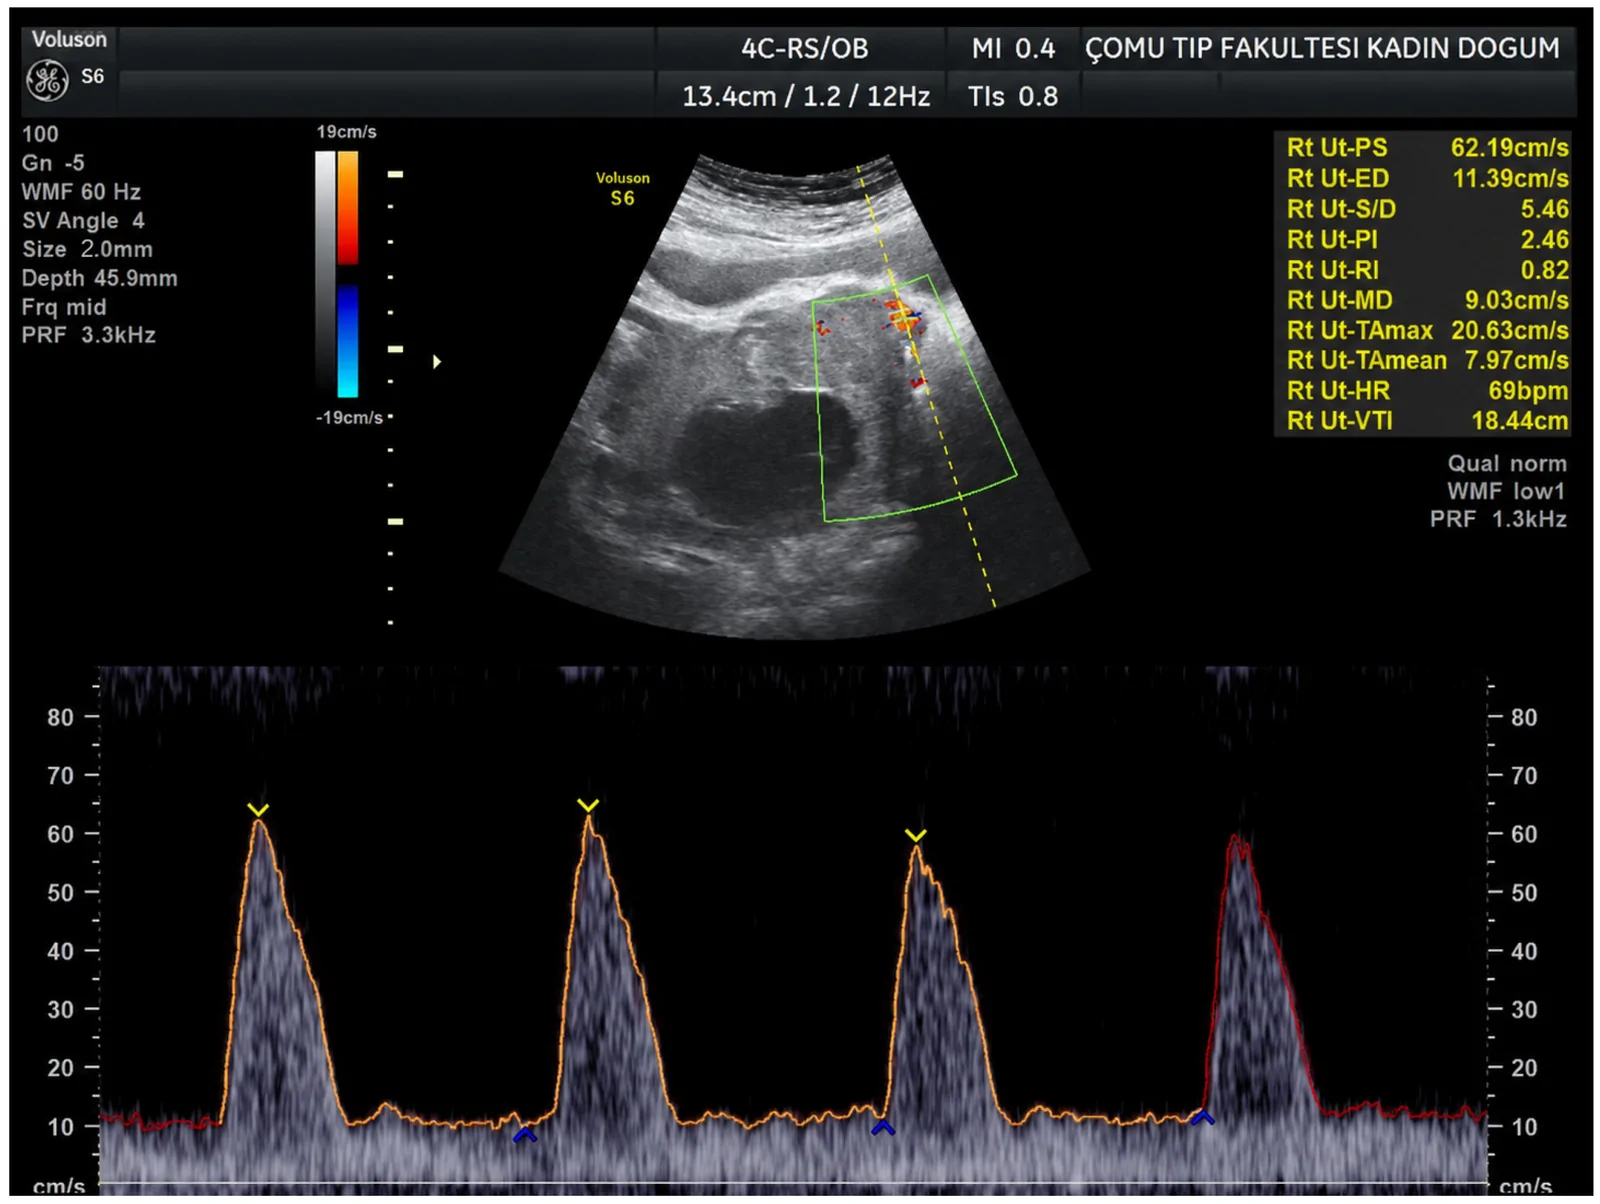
Representative bilateral uterine artery Doppler examination performed according to the Fetal Medicine Foundation protocol. The three orange-traced waveforms represent the measured cycles used for pulsatility index calculation; the additional (red-traced) waveform is an adjacent, unmeasured cardiac cycle automatically displayed by the ultrasound system. The green box denotes the Doppler sample gate positioned over the uterine artery, the yellow dashed line indicates the angle-correction line used to align the insonation angle with the vessel, and the yellow and blue chevrons mark the automatically identified systolic peak and end-diastolic points, respectively, used for pulsatility index calculation.
